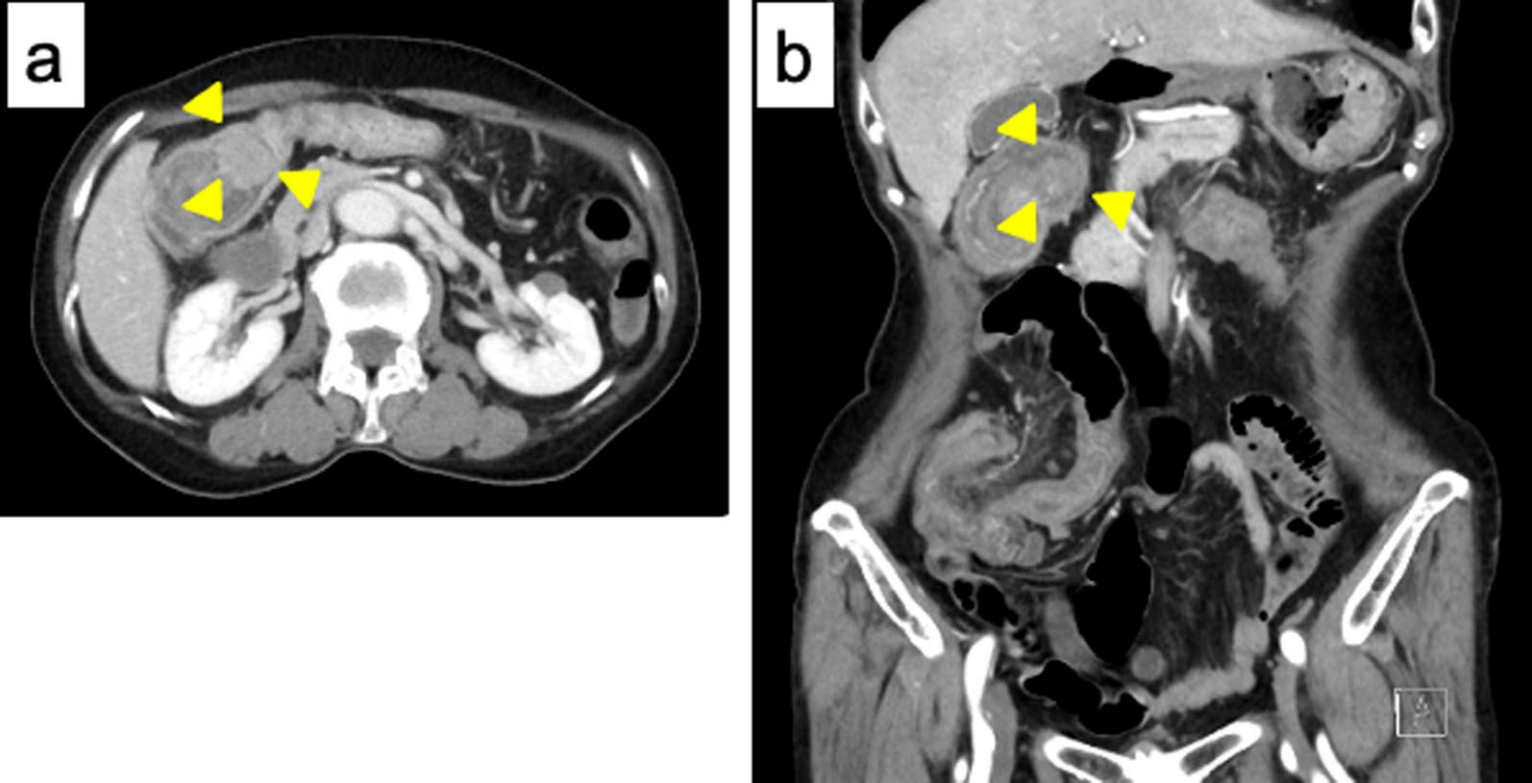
An abdominal contrast CT scan. Target sign is detected in the ascending colon in right upper abdomen, which indicates intussusception. a Axial views. b coronal views. Arrowhead: a lead point
